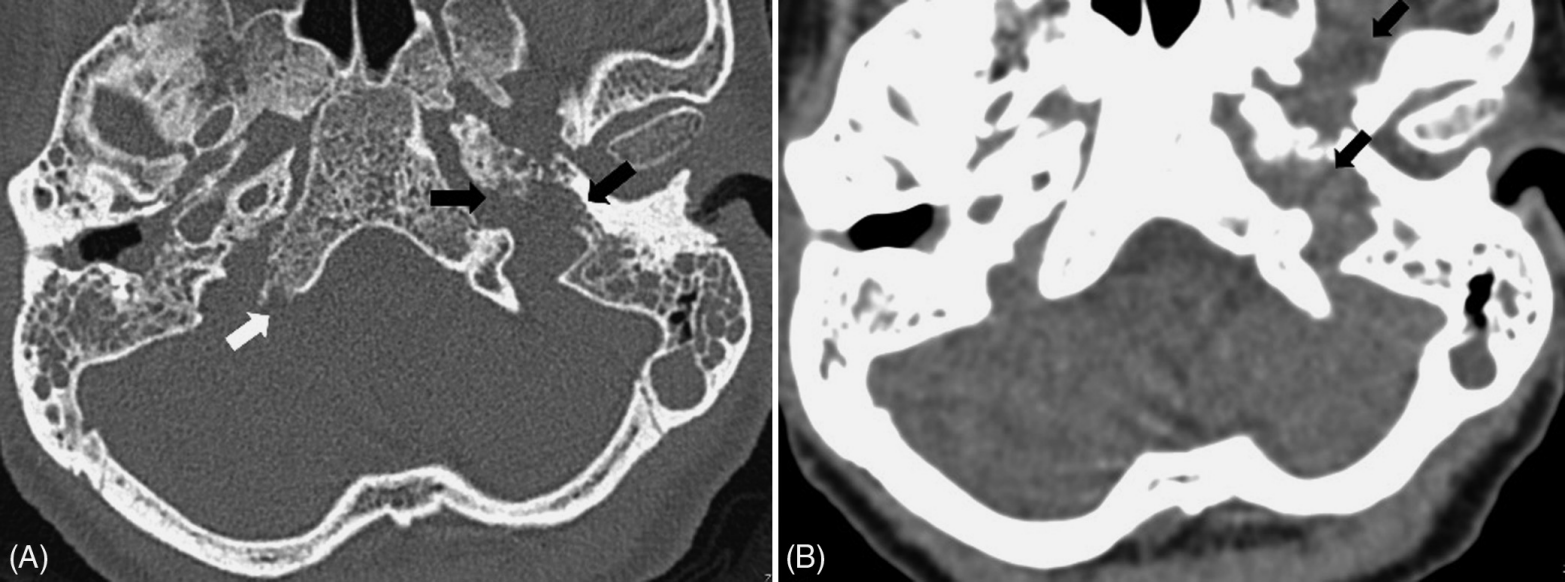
Axial computed tomography bone (A) and soft tissue window (B) images demonstrate opacification of the bilateral mastoid air cells and right middle ear cavity, compatible with otomastoiditis. Image A shows erosive changes in the right posterior clivus (white arrow) and left petrous apex and jugular foramen (black arrows). Image B shows abnormal soft tissue seen in the left jugular foramen and masticator space (black arrows).
